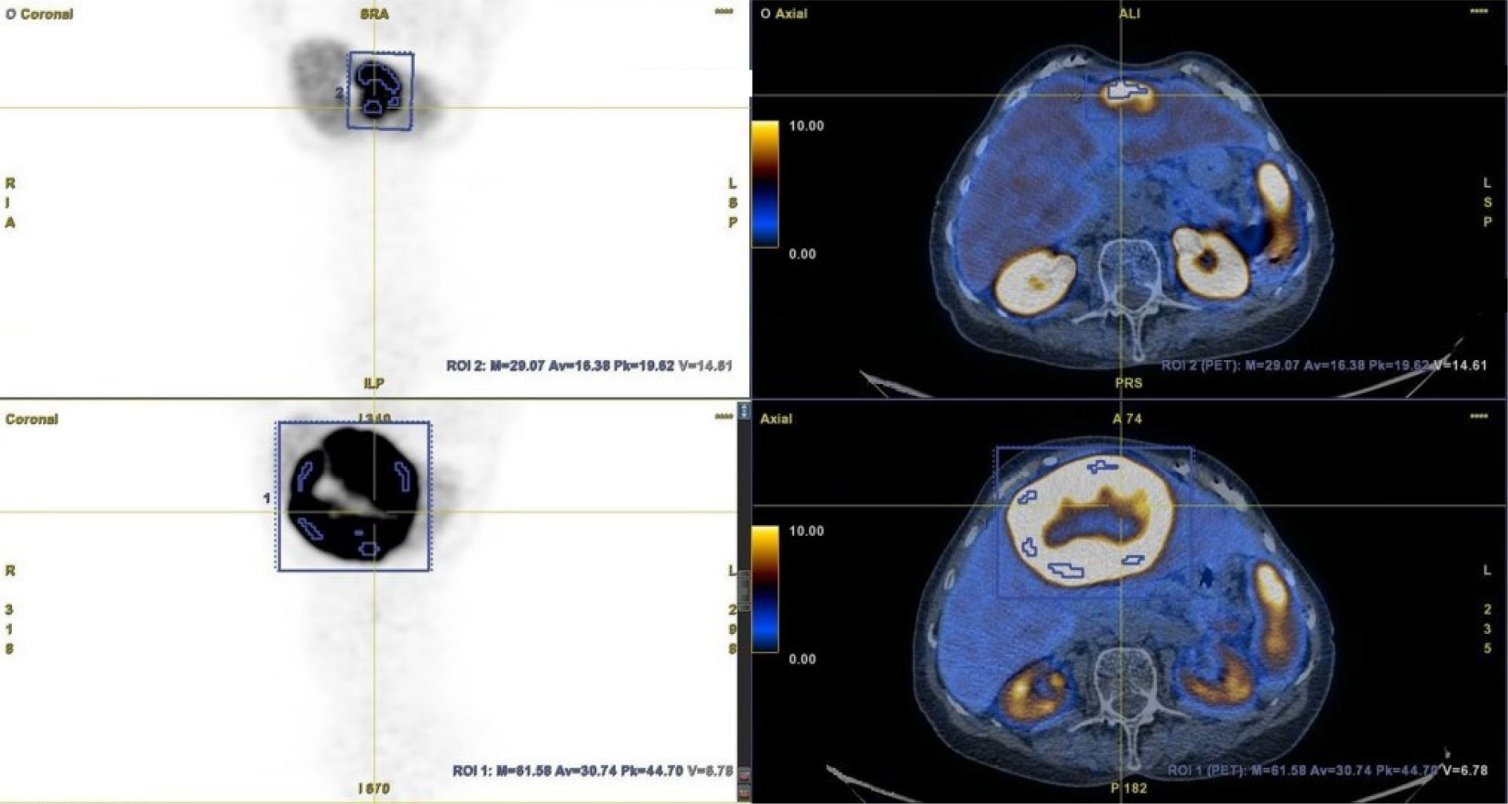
Method of metastatic lesion segmentation. [68Ga]Ga-DOTA-TATE PET/CT before PRRT (bottom row) and after PRRT (upper row).
